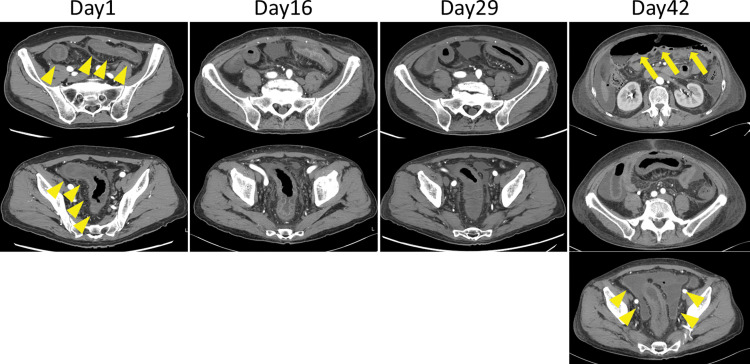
Abdominal CT findings. Day 1: Circumferential wall thickening from the descending colon to the upper rectum (arrowheads). Days 16, 29: No clear improvement is observed. Day 42: The contrast enhancement of the sigmoid colon is weak with free intraperitoneal air (arrows) and ascites (arrowheads).
